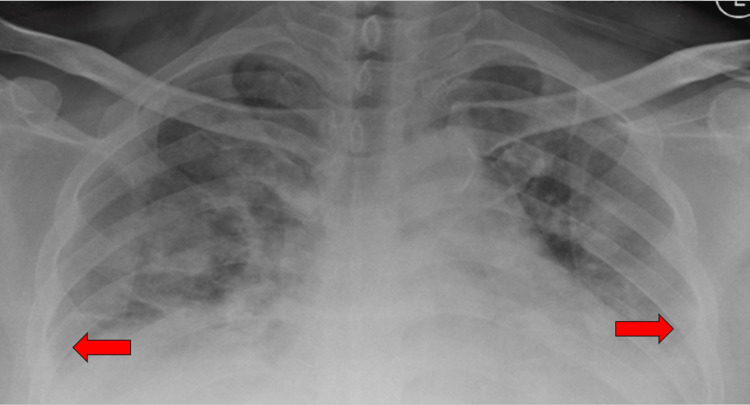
Chest x-ray showing bilateral blunted costophrenic angles with pneumonic changes.
